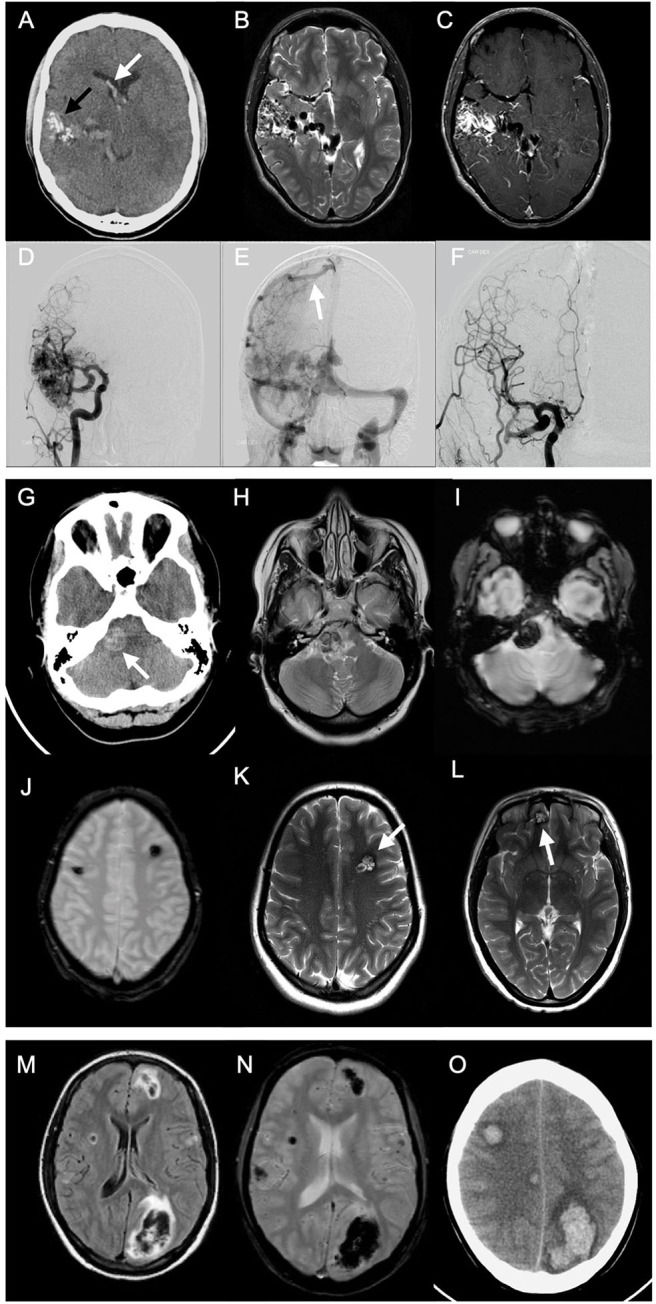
Multimodality imaging of different spontaneous intracerebral hemorrhage etiologies in young adults. *Case 1*: A 20-year-old male presented to emergency with sudden onset headache. Emergent CT was done. Axial non-enhanced CT (NECT) (A) shows right temporal serpentine calcifications (*black arrow*), associated with intraventricular hemorrhage (*white arrow*) and calcifications. Axial T2-weighted images (T2WI) (B) show classic wedge-shaped arteriovenous malformation (AVM) with flow voids representing feeding arteries and dilated draining veins. Axial T1-weighted images (T1WI) with contrast (C) show avid AVM nidus enhancement. Pre- (D,E) and postoperative (F) catheter angiography shows dilated middle and posterior cerebral arteries feeding AVM and dilated draining cortical vein to superior sagital sinus (*white arrow*). *Case 2*: A 24-year-old female, presented with history of headache persisting for 1 week. NECT was performed (G) showing hyperdense lesion at the right cerebellopontine angle. Axial T2WI (H) show hypointense lesion with surrounding edema. T1WI with contrast showed no contrast enhancement (not shown). T2*gradient echo (GRE) scan (I) shows “blooming” effect within the lesion. Another T2*GRE image (J) at different levels and T2WI (K,L) showing multiple lesions at different sites with typical “popcorn” appearance, representing multiple cavernomas. *Case 3*: A 20-year-old female with history of headache for 1 week. Emergent MRI scan was performed. Fluid-attenuated inversion recovery (FLAIR) images (M) show multifocal areas of hypointensities, mainly lobar in location, surrounded by hyperintense margins representing edema. T2*GRE image (N) shows “blooming” representing multiple acute intracerebral hemorrhages (ICHs). Two days later, follow-up NECT (O) showed multiple ICHs with surrounding moderate edema. Investigations revealed acute leukemia as underlying etiology.
